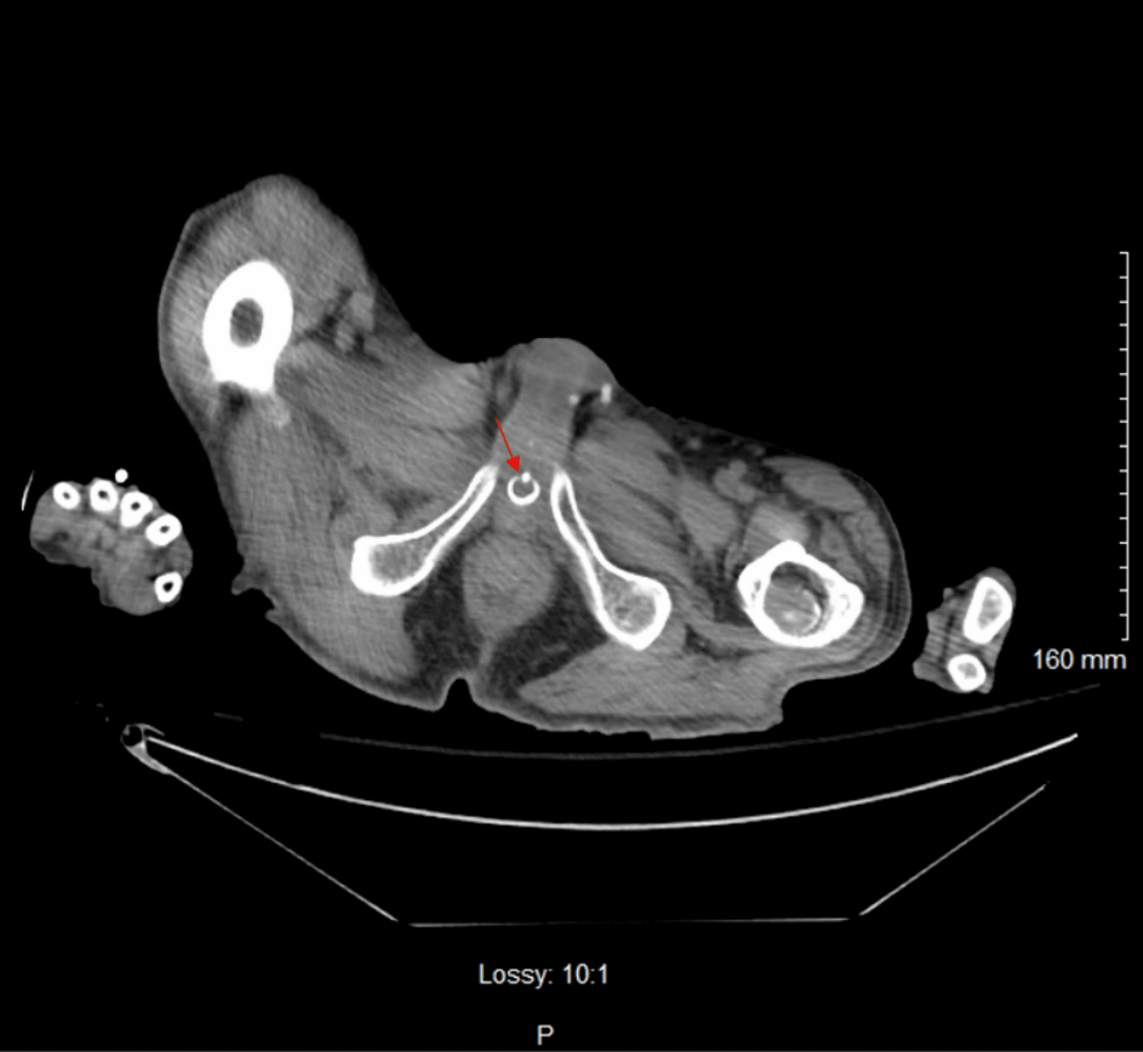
Axial non-contrast CT image at the prostate and penile region The prostate appears enlarged with multiple brachytherapy seeds and prior transurethral resection of the prostate (TURP). The red arrow points to the internal bladder-urethral sphincter.
